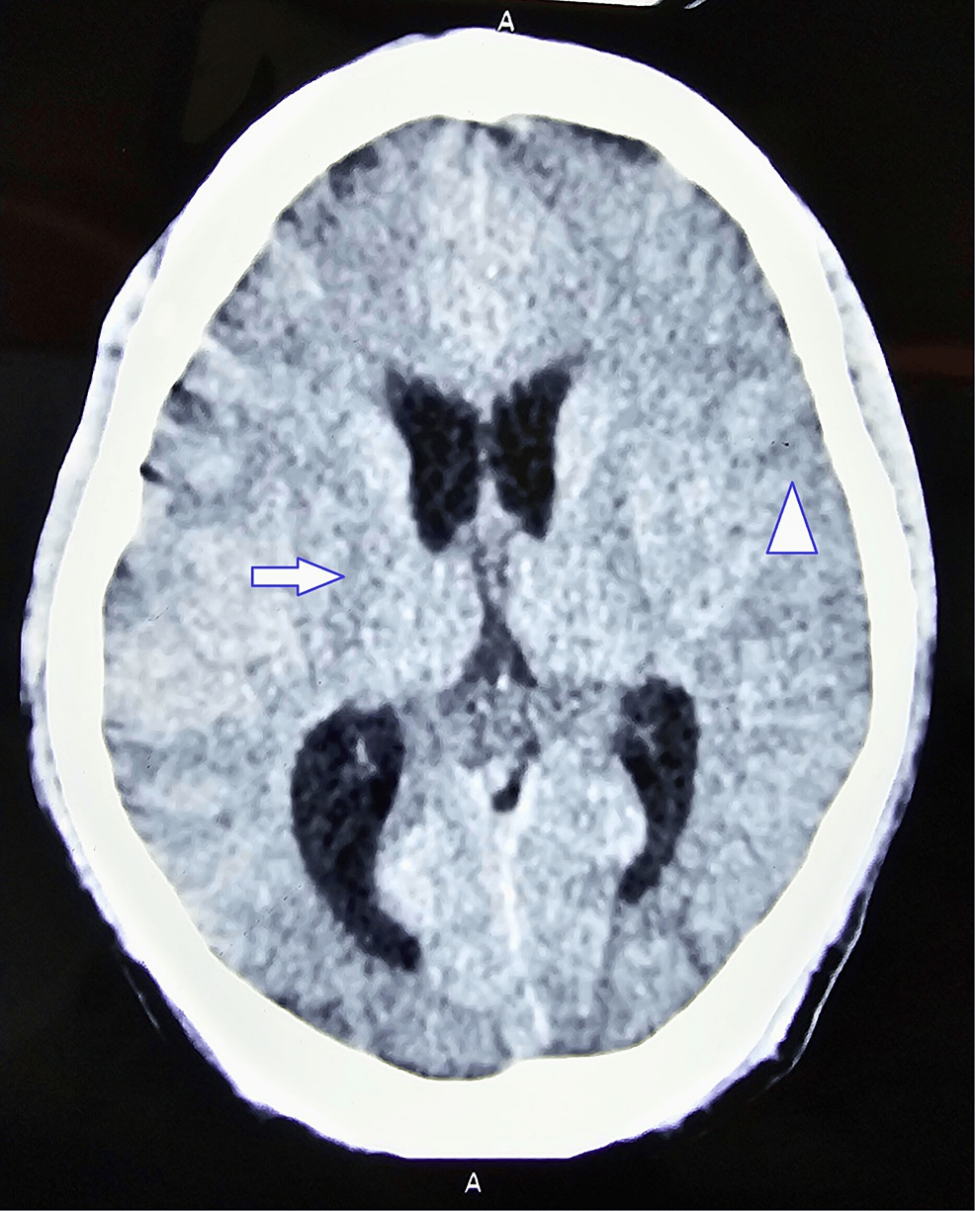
Non-contrast computed tomography head showing cerebral edema Arrow showing poor grey-white differentiation. Arrowhead showing obliteration of sulci. Both features of cerebral edema.
